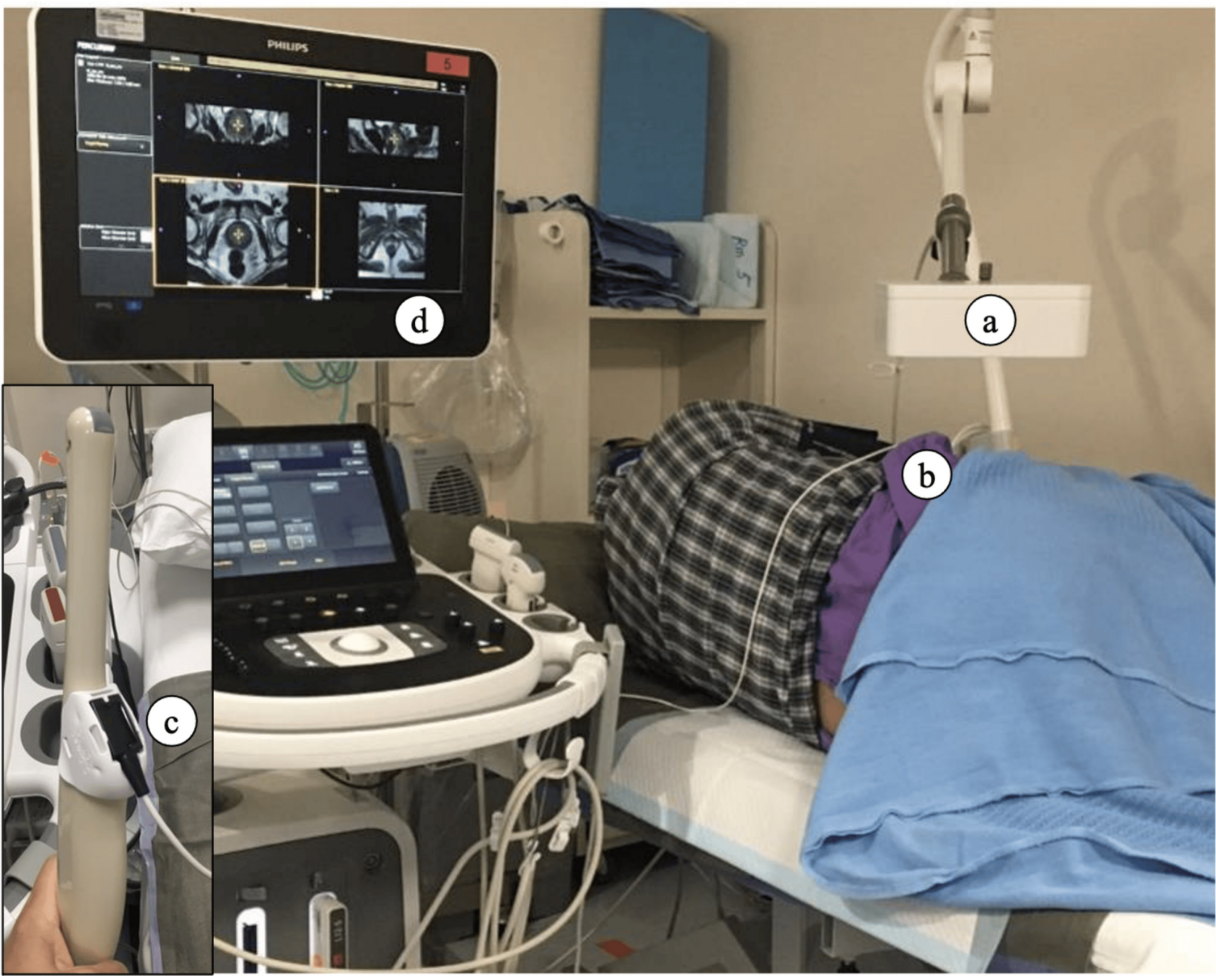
Electromagnetic tracking system setup (a) electromagnetic field generator; (b) patient tracker secured on the patient; (c) endocavity ultrasound tracker attached to the ultrasound transducer via a tracking bracket; (d) ultrasound machine with connection unit and MRI data uploaded. Abbreviations: MRI=magnetic resonance imaging
